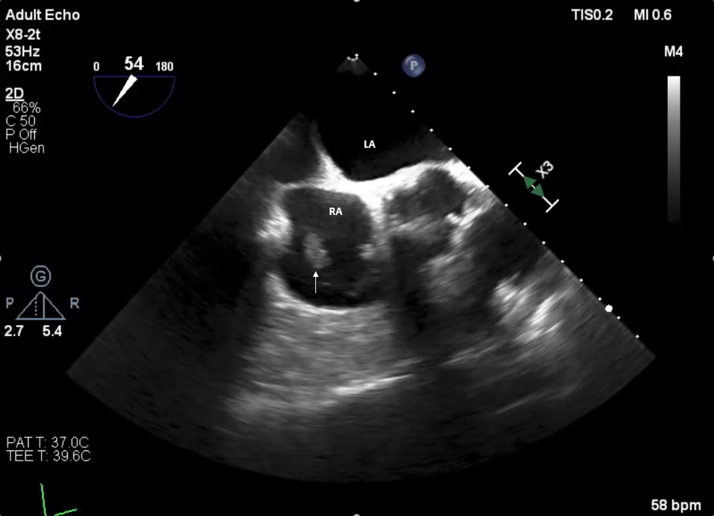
Transesophageal Echocardiography Within 6 Hours After Procedure Transesophageal echocardiography showing significant decrease in the number and size of tricuspid vegetation (arrow). LA = left atrium; RA = right atrium.
